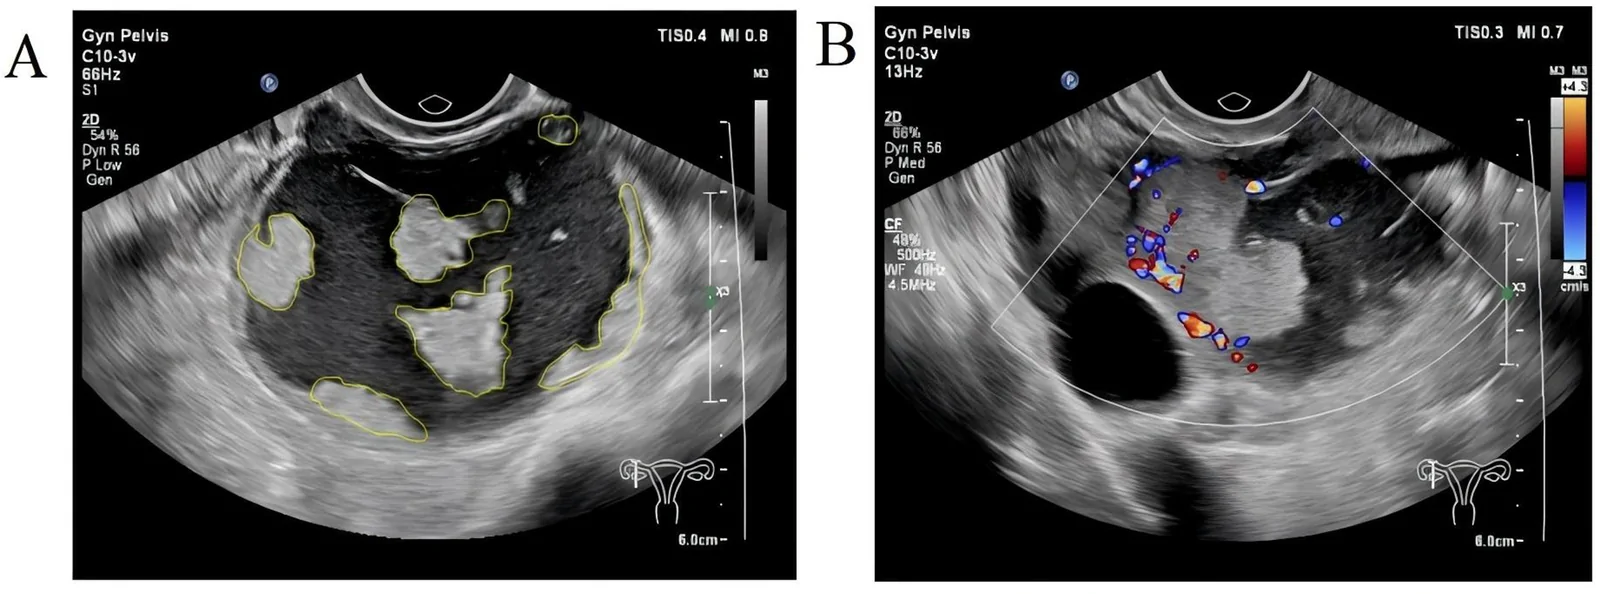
Serous borderline tumor of the right ovary, with dimensions of 6.9 cm × 4.5 cm × 5.8 cm. (A) Grayscale ultrasound demonstrates a complex cystic-solid mass. Multiple solid components are outlined by the yellow lines. (B) Color Doppler image showing internal vascularity within the solid component. Sonographer A classified it as O-RADS category 5, while Sonographer B also classified it as O-RADS category 5.
